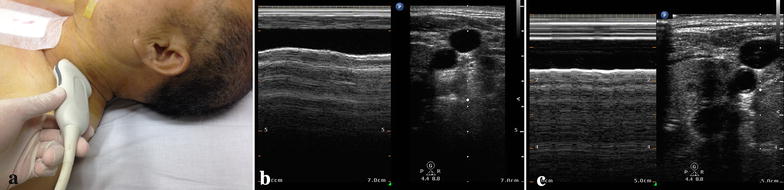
Ultrasound probe position for IJV detection at the cricoid cartilage level (a). The patient is in the supine position at 30°. M-mode assessment of the antero-posterior diameter of the IJV in a responsive patient (b, a high variability of IJV diameter is seen) and in a non-responsive patient (c, lack of variation of the IJV diameter is seen) while on mechanical ventilation
